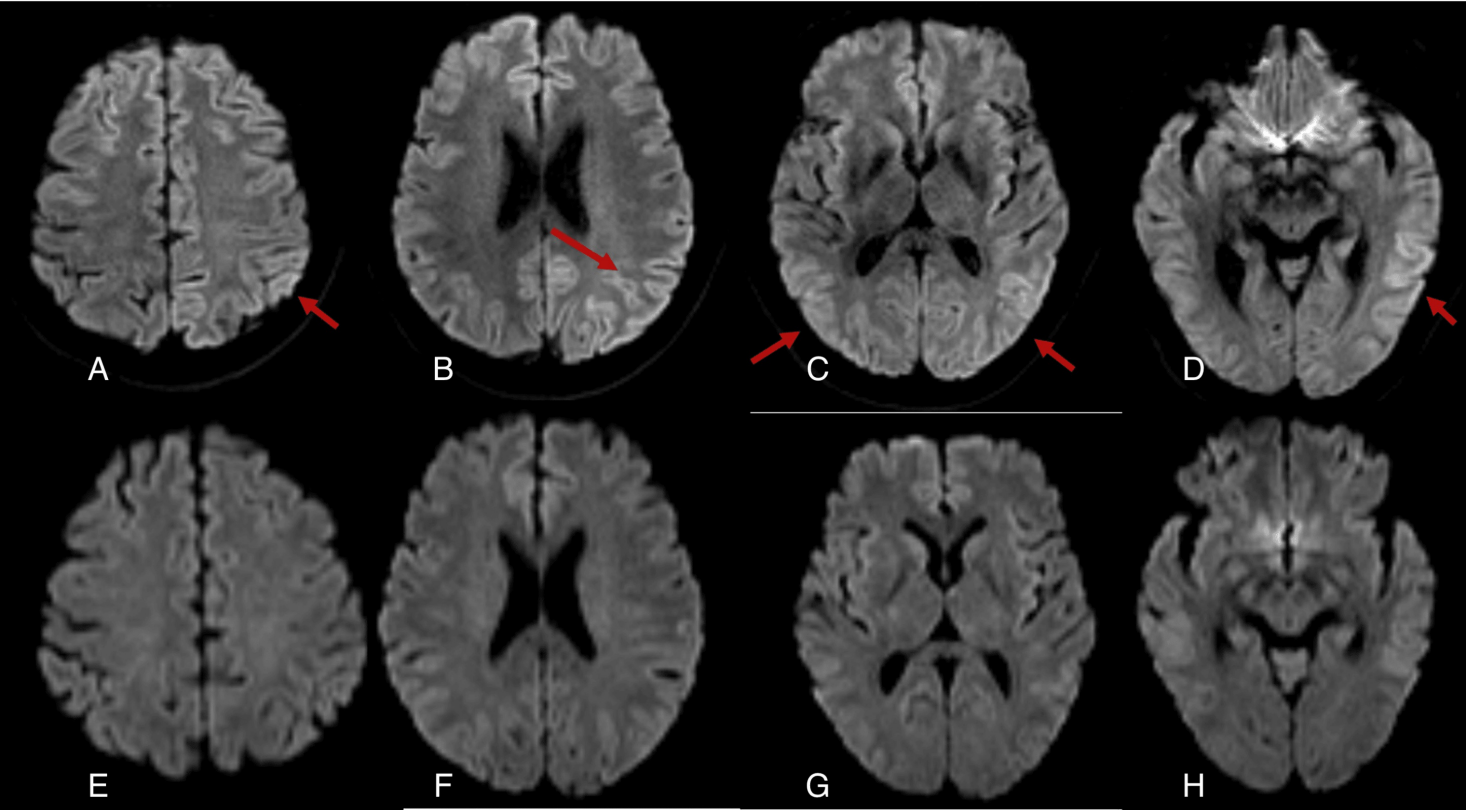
Axial diffusion-weighted imaging (DWI) of the brain (A–D) Acute-phase axial DWI MRI: (A) Subtle diffuse cortical hyperintensity, more apparent in the left parietal cortex; (B) Persistent asymmetric cortical hyperintensity involving the left parietal convexity; (C) Continued cortical signal abnormality at a slightly lower axial level, still predominantly left-sided; (D) Inferior axial section showing mild cortical hyperintensity in the left temporo-occipital region. (E–H) Follow-up MRI performed four days later, demonstrating complete resolution of the previously noted cortical signal abnormalities. (E) Normal cortical signal at the level corresponding to (A).
